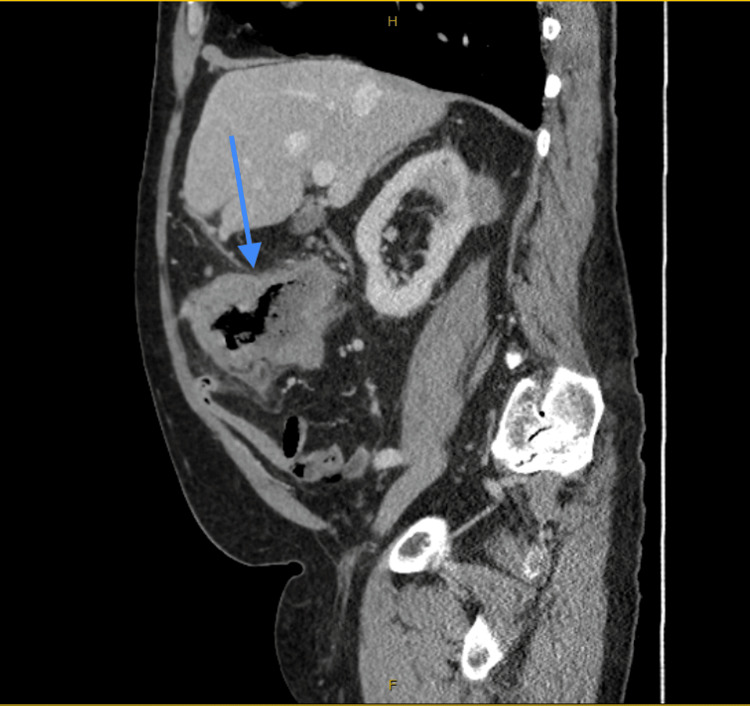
CT abdomen and pelvis with intravenous contrast (sagittal view) demonstrating significant irregular thickening within a 10 cm segment of the proximal transverse colon (arrow).
